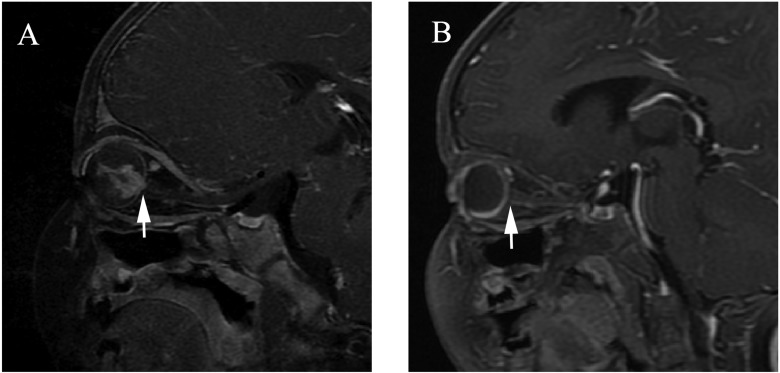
Clinically diagnosed retinoblastoma in a 16-month-old male (patient 3) Oblique sagittal contrast-enhanced T1-weighted, fat-saturated MRI shows abnormal nodular enhancement (arrow) of the postlaminar optic nerve (A), a finding indicative of postlaminar optic nerve invasion. After three cycles of intra-arterial chemotherapy (IAC), the abnormal nodular enhancement of the postlaminar optic nerve disappeared (B).
